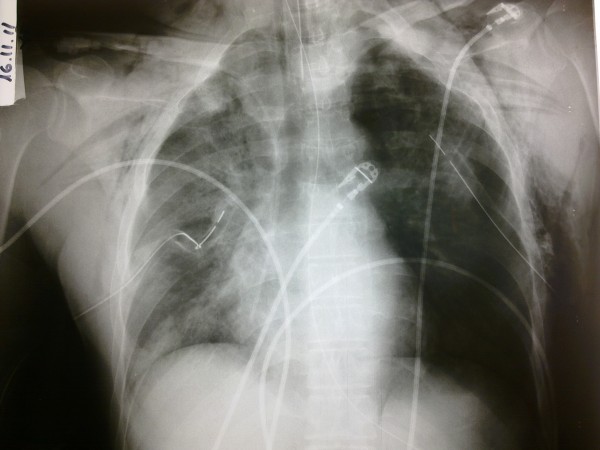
Chest X-ray taken after tube thoracostomies were inserted. Note multiple rib fractures, subcutaneous emphysema, multiple lung opacities, particularly on the right, corresponding to sites of lung contusion and residual pneumothorax on the left side.
